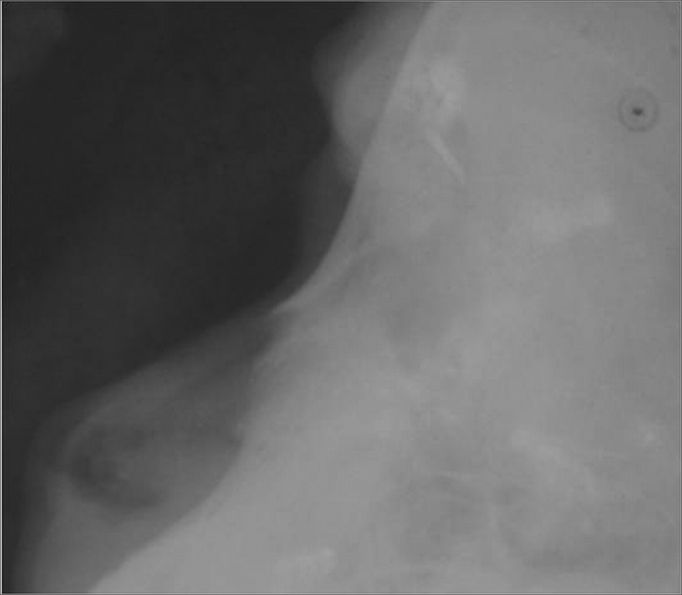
Left side lateral radiograph of the nose in the preoperative
